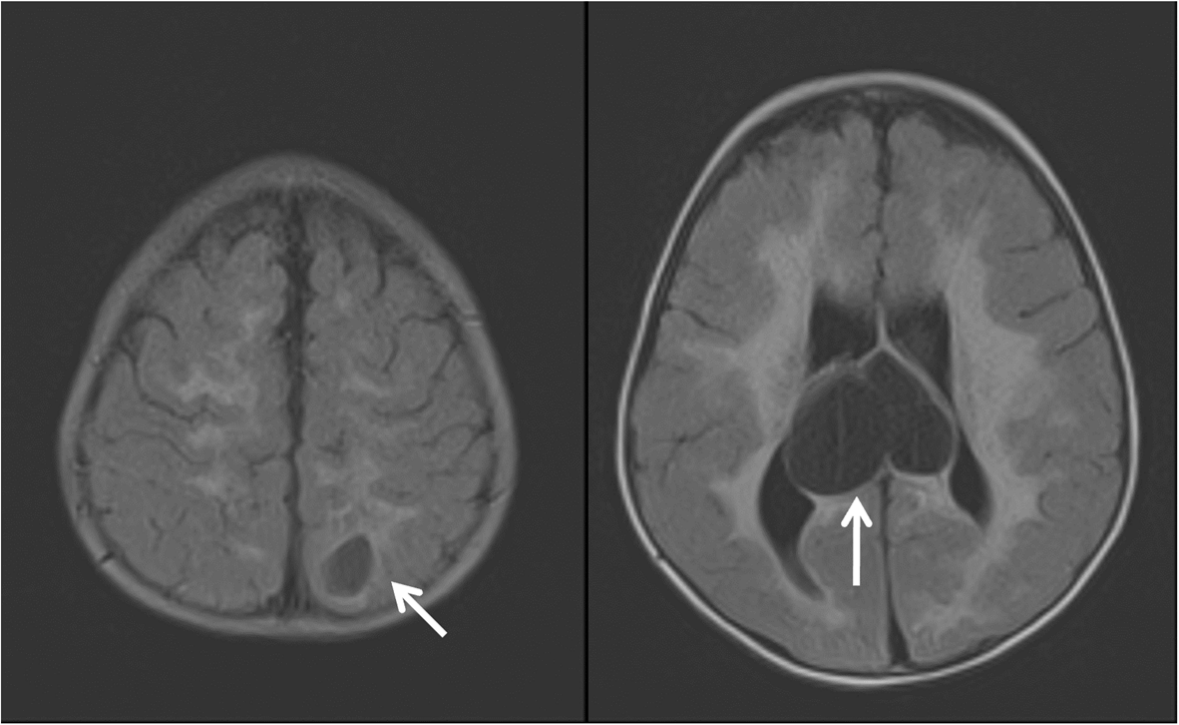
Intracranial cysts in cranial MRI
